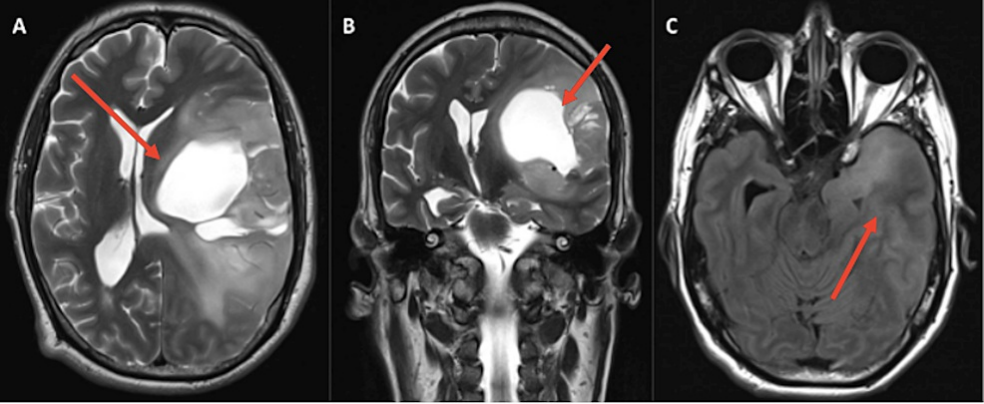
T2-weighted cranial MRI A (axial) and B (coronal): T2-weighted, cystic behavior, perilesional hyperintensity corresponding to tumor infiltration. Frontal-parietal-temporal lesion. C: Flair sequence, showing perilesional edema in the temporal pole and in the mesial temporal region.
